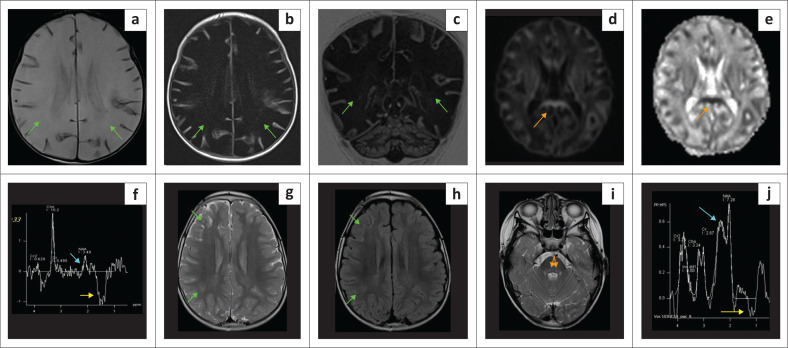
(a-f) A 15-day-old male with *ornithine transcarbamylase (OTC) deficiency*. The MRI reveals restricted diffusion in the corpus callosum (orange arrow), diffuse white matter interstitial oedema (green arrows), corresponding T1-W signal prolongation, with a glutamine-glutamate peak on the upstroke of the N-acetylaspartate peak (cyan arrow) and lactate peak (yellow arrow). Note the exaggerated choline: N-acetylaspartate ratio for neonates. (g-j) A 5-year-old male with a family history of a male sibling who passed away with a similar disorder. *Ornithine transcarbamylase deficiency* with subtle subcortical white matter changes (green arrows) with a glutamine-glutamate peak on the upstroke of the N-acetylaspartate peak (cyan arrow), lactate peak (yellow arrow) and central tegmental tract (orange arrows) hyperintensity.
